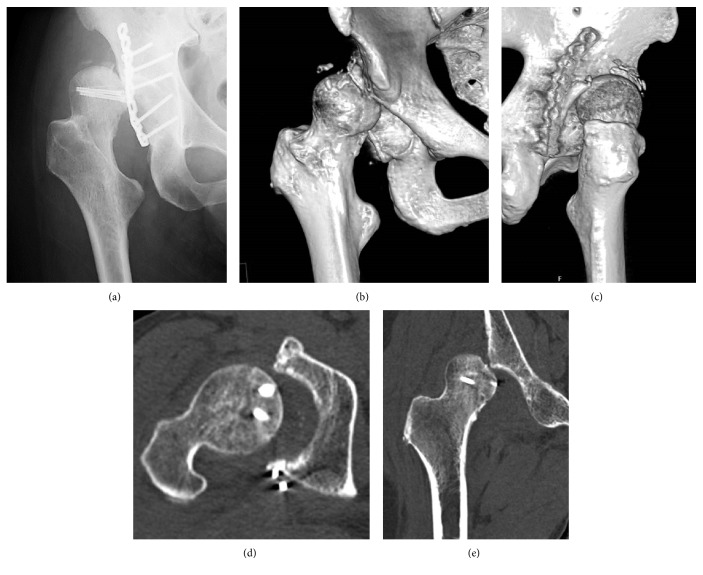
Case 1. (a) Anteroposterior X-ray image of the right hip at the superolateral dislocation. (b) Anteroposterior three-dimensional computed tomography showing superolateral dislocation of the femoral head and a bony defect of the superior part of the acetabular rim. (c) Posteroanterior three-dimensional computed tomography showing no displacement of the posterior fragments or implant failures. (d) Axial computed tomography showing irregular congruity, no displacement of the femoral head or posterior wall. (e) Coronal computed tomography showing superolateral dislocation of the femoral head and no displacement of the femoral head.
